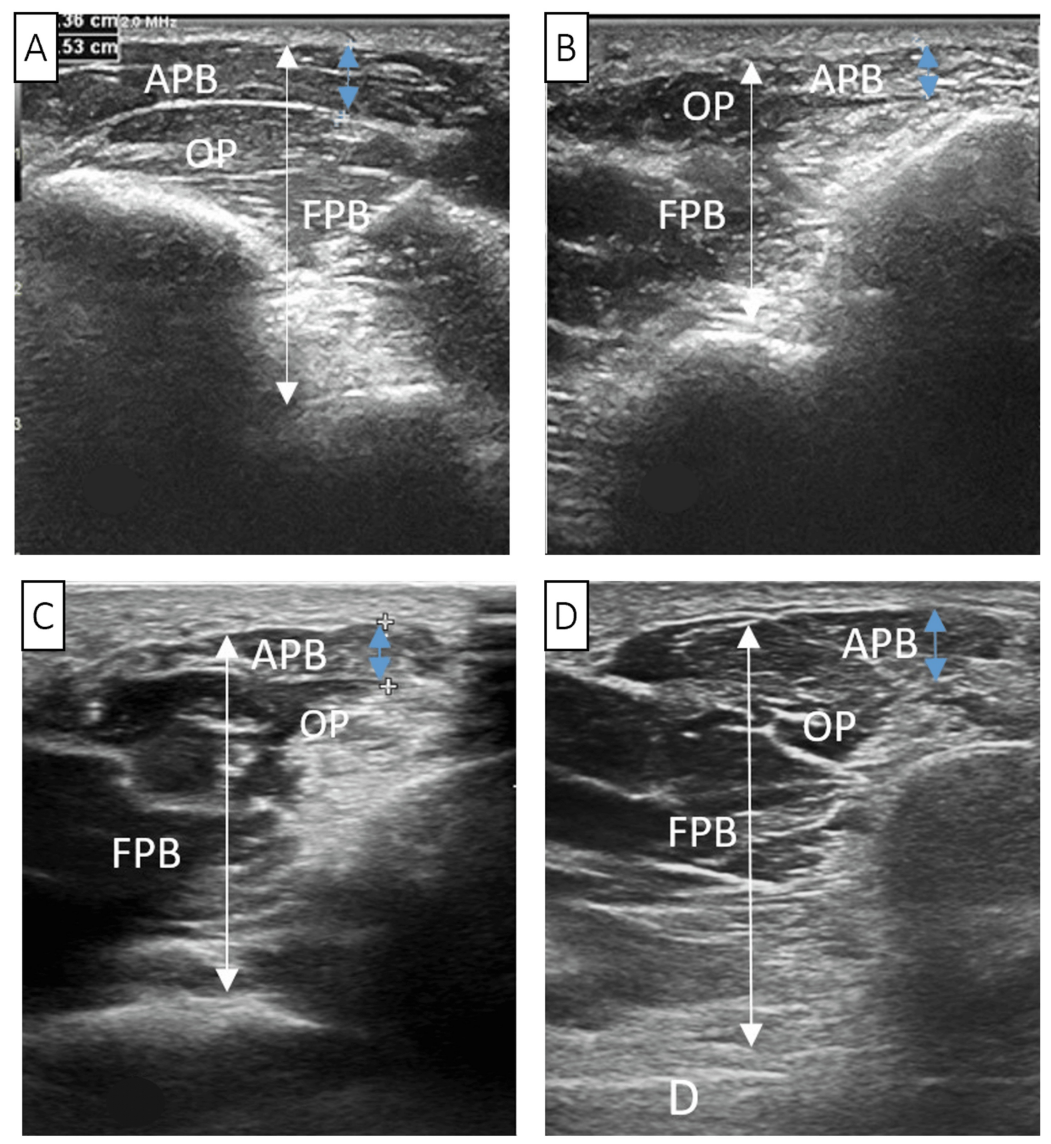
Ultrasound imaging of the muscles of the right (A, normal imaging) and left (B, C, D) thenar eminence of the thumb at 7 (B), 17 (C), and 21 (D) months of the disease. The ultrasound probe is positioned over the thenar region, perpendicular to the first metacarpal bone. The white arrows indicate the total thickness of the thenar muscles, and the blue arrows indicate the thickness of the APB. APB: abductor pollicis brevis; OP: opponens pollicis; FPB: flexor pollicis brevis
